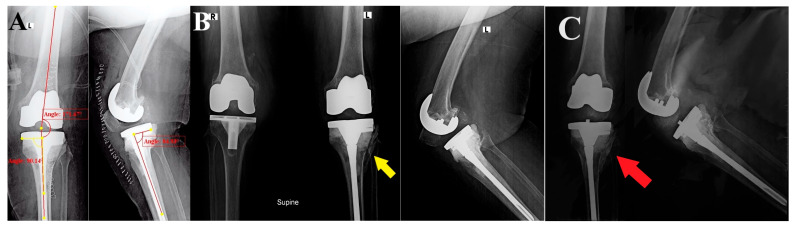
Postoperative and follow-up radiographs: (A) Immediate AP and lateral views of the revised TKA show the reconstruction of the lateral tibial defect, the restoration of the lower limb alignment, and proper implant positioning. (B) At eight weeks of follow-up, the limb and implant alignment was maintained, and the lateral tibial plateau cortex fracture showed signs of healing and callus formation (yellow arrow). (C) At six months of follow-up, the fracture showed healing (red arrow) and stable implants.
